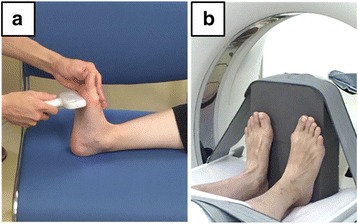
Foot position for ultrasonography (US) and computed tomography (CT). The ankle, proximal interphalangeal joint, and metatarsophalangeal joints are in a neutral position in US and CT scans. a The US probe was attached to the landmark line at right angles to the plantar surface. b The foot position was fixed using a footboard on the bed of the CT scanner
